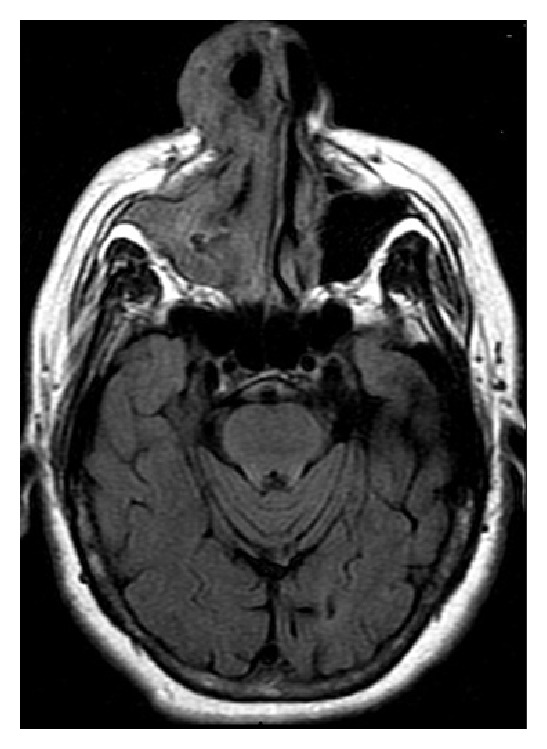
Axial MRI T2 FLAIR image of the nasal lesion with relatively homogeneous, low intensity signal.
